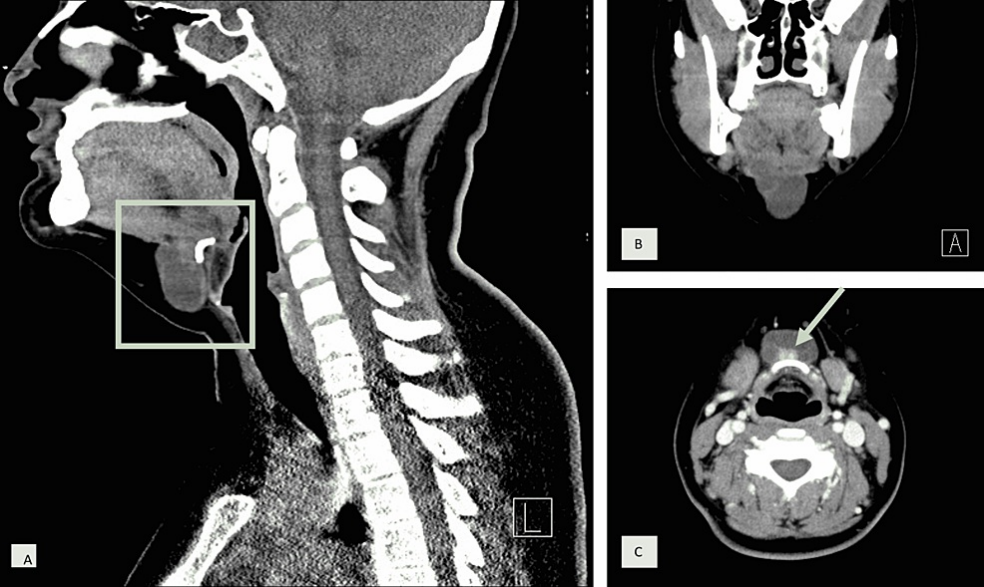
Cervical CT-angiogram A: Sagittal plane revealing cystic mass adjacent to the hyoid bone (rectangle), B: Coronal plane with the thyroglossal cyst extending to the base of the tongue, C: Axial plane showing cystic multiseptated mass anterior to the hyoid bone; solid intracystic component (arrow)
